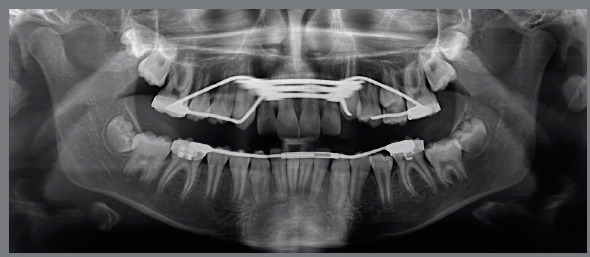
Progress panoramic radiograph.
